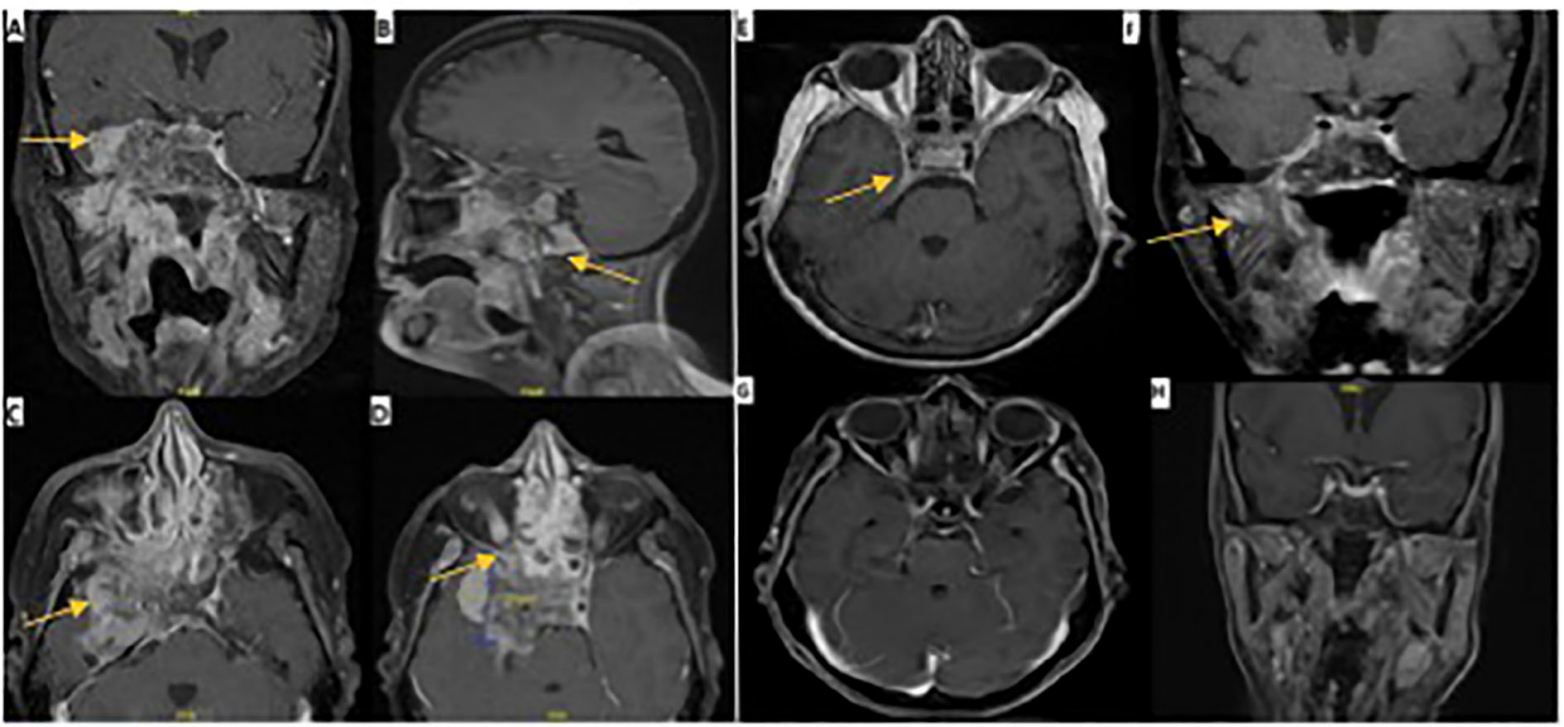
MRI images pre and post-CRT. Pre-ICT: (A) axial, coronal, and sagittal views of T1 with contrast MRI brain showing an aggressive mass invading the temporal lobe intracranially. (B) extension to the skull base. (C) extension to cavernous sinus. (D) extension to superior orbital fissure. One month post-ICT: (E, F) demonstrate axial and coronal views of T1 with contrast 4 months post-ICT with significant regression of tumor size and few remnants as indicated by the arrows. Eight months post-CRT: (G, H) demonstrate axial and coronal views of T1 with contrast with almost complete resolution of tumor.
